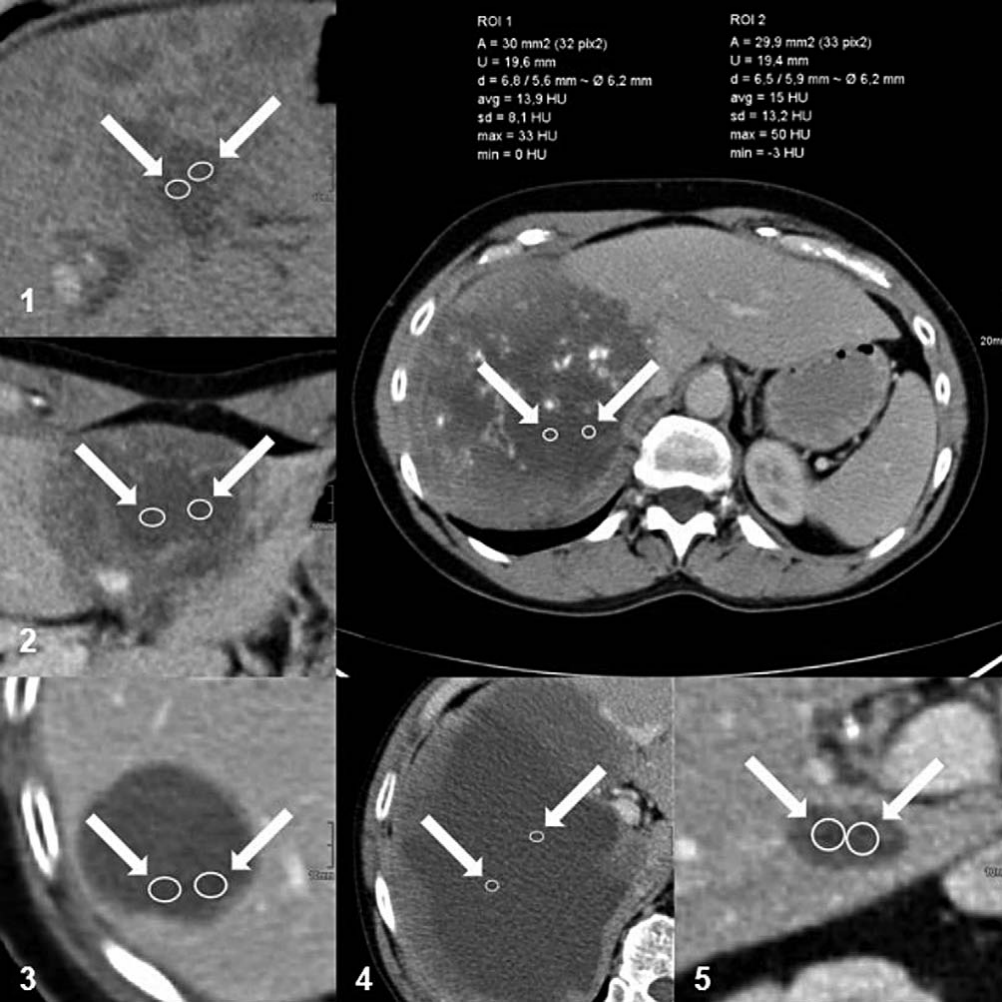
Example of density measurement in the cystoid portions of AE lesions on CT. Left and below: in each case, two enlarged ROIs within the cystoid portions of AE lesions of different primary morphological types: (1) Type I “diffuse infiltrating with cystoid portion”; (2) Type II “primarily circumscribed tumour-like with cystoid portion”; (3) Type IIIa “primarily cystoid intermediate without solid portion at the edge”; (4) Type IIIb “primarily cystoid widespread with solid portion at the edge”; (5) Type IV “small cystoid/metastatic”. Above right: two ROIs with surface data and HU values (max, min, mean and standard deviation) within the cystoid portion of an AE lesion involving both lobes of the liver. (AE = alveolar echinococcosis; CT = computed tomography; HU = Hounsfield unit; ROI = region of interest).
